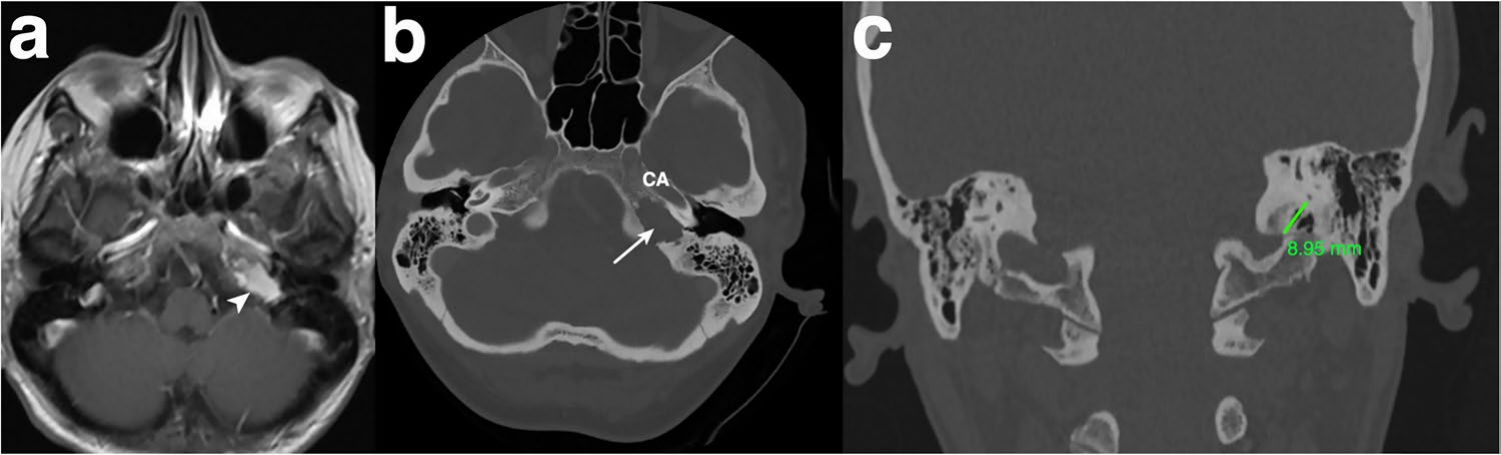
Evaluation of the feasibility of the TI-A for accessing an intrapetrous extradural lesion at the petrous apex (PA) and petroclival junction (PJ) a Preoperative T1-weighted axial contrast-enhanced magnetic resonance imaging (MRI) and b axial high-resolution bone window computed tomography (CT) scan are presented, showing a contrast-enhancing lesion (white arrowhead) with osseous erosion (white arrow). c The measured horizontal distance of 8.95 mm on the coronal cranial CT indicates adequate infralabyrinthine access
